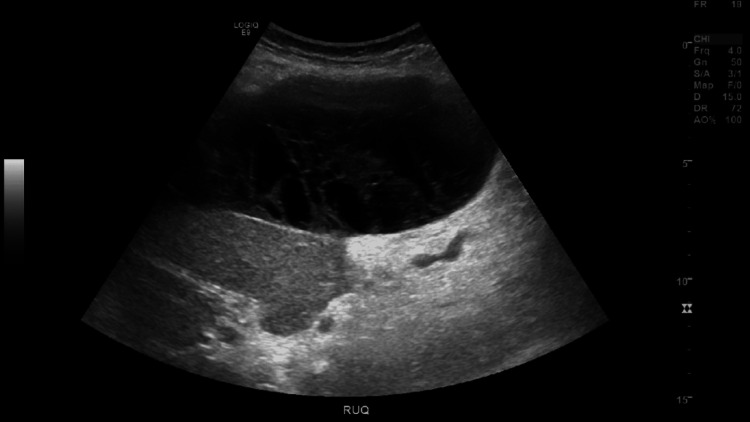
RUQ ultrasound showing hepatic subcapsular collection with multiple loculations. RUQ: right upper quadrant
